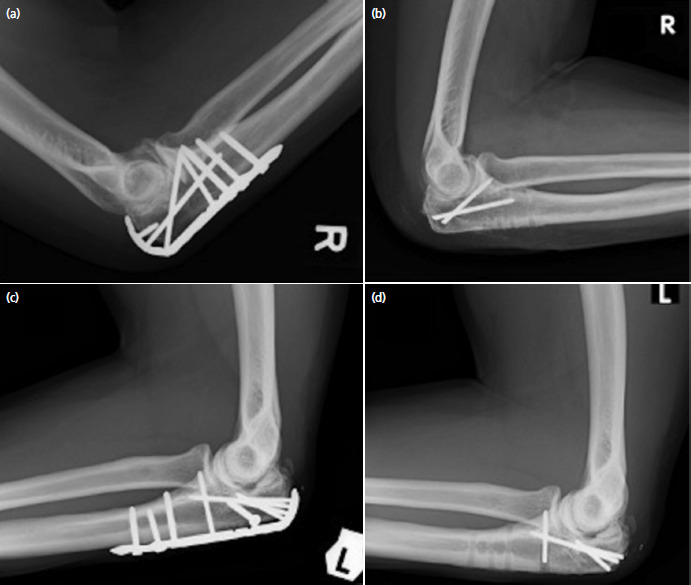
Two cases with broken screws. (a) Pre-operative radiograph showing a healed olecranon fracture with a locking plate in situ. (b) Post-operative radiograph showing two broken and retained locking screws. (c) Pre-operative radiograph of another patient showing a healed olecranon fracture with a locking plate in situ. (d) Post-operative radiograph showing four broken locking screws.
